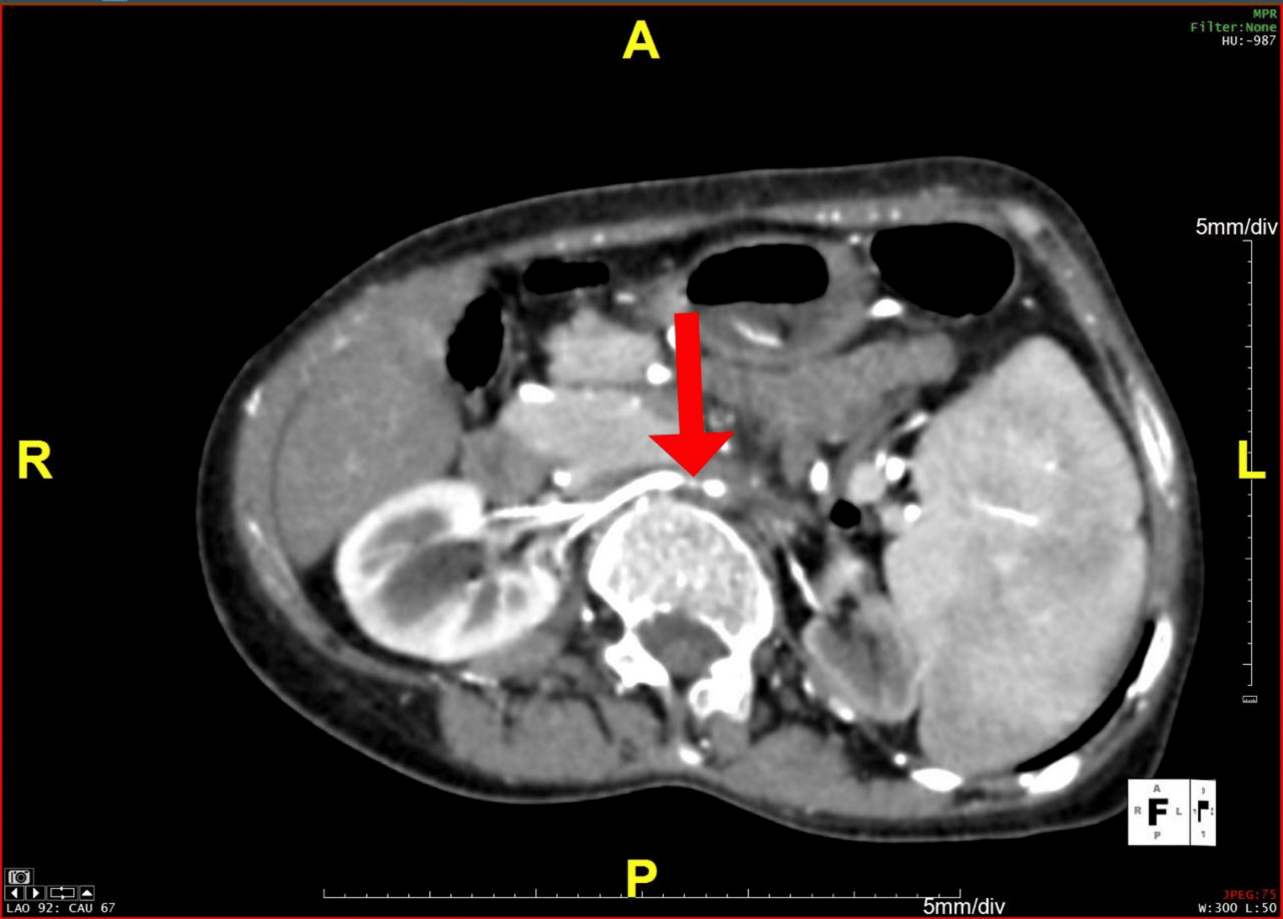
An axial section of the CT angiogram demonstrating stenosis of right renal artery at its origin CT: computed tomography
